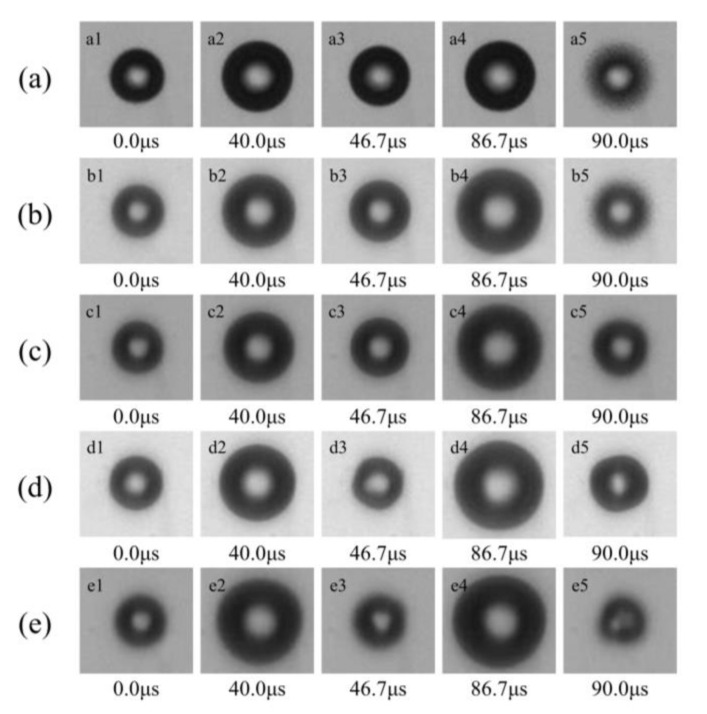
Temporal evolutions of the single bubble in free-field exposed to ultrasound in (a) Liquid A (surface tension *σ* = 72.59 mN/m); (b) Liquid B (surface tension *σ* = 58.75 mN/m); (c) Liquid C (surface tension *σ* = 49.34 mN/m); (d) Liquid D (surface tension *σ* = 38.47 mN/m); (e) Liquid E (surface tension *σ* = 27.13 mN/m). (*R*_0_ = 20 μm, T = 21 °C).
